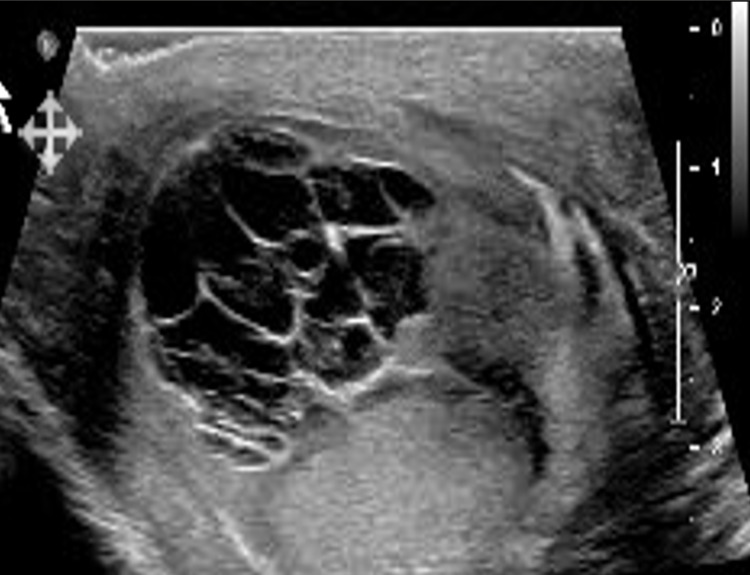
Ultrasound scan of left testicle revealing 2 cm extra-testicular lesion with multiple fluid-filled loculations
